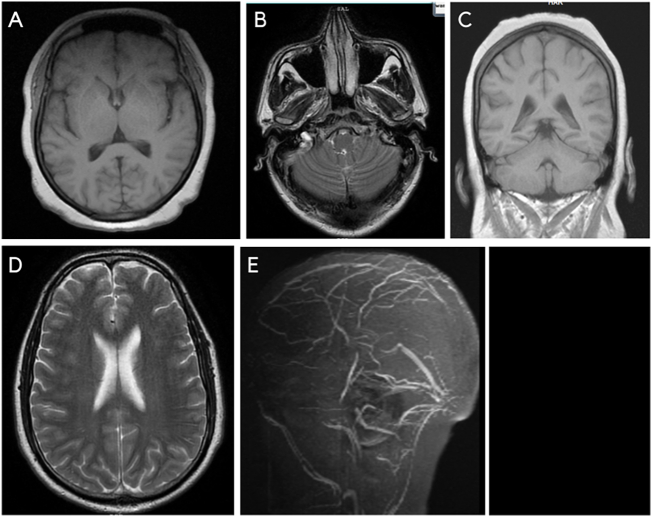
A-E: Magnetic resonance imaging (MRI) and magnetic resonance venography (MRV) images showing extensive cerebral venous sinus thrombosis, including the superior sagittal sinus and bilateral lateral and sigmoid sinuses.
